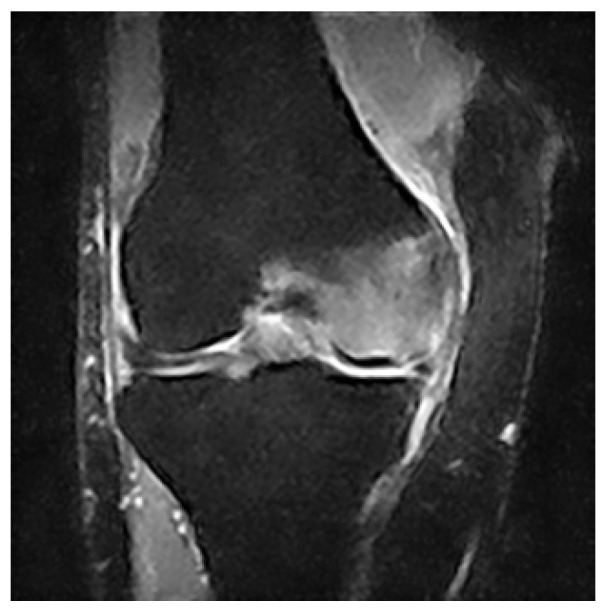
Coronal MRI image showing a typical BME pattern of our patients.
